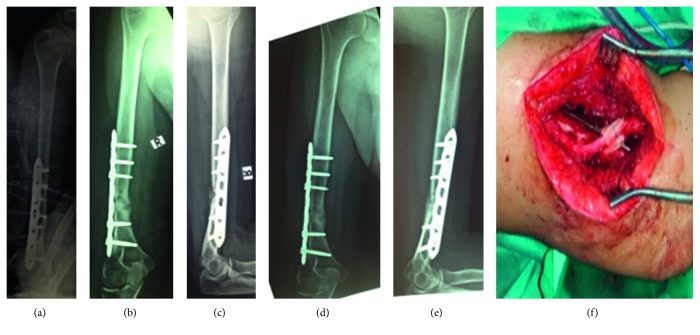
(a) X-ray after the initial treatment of the humerus fracture with a dynamic compression plate. (b and c) X-ray after the nonunion treatment. (d and e) X-ray 3 months postoperatively showing callus formation in the previous nonunion site. (f) Intraoperative picture after the nonunion treatment showing the radial nerve.
